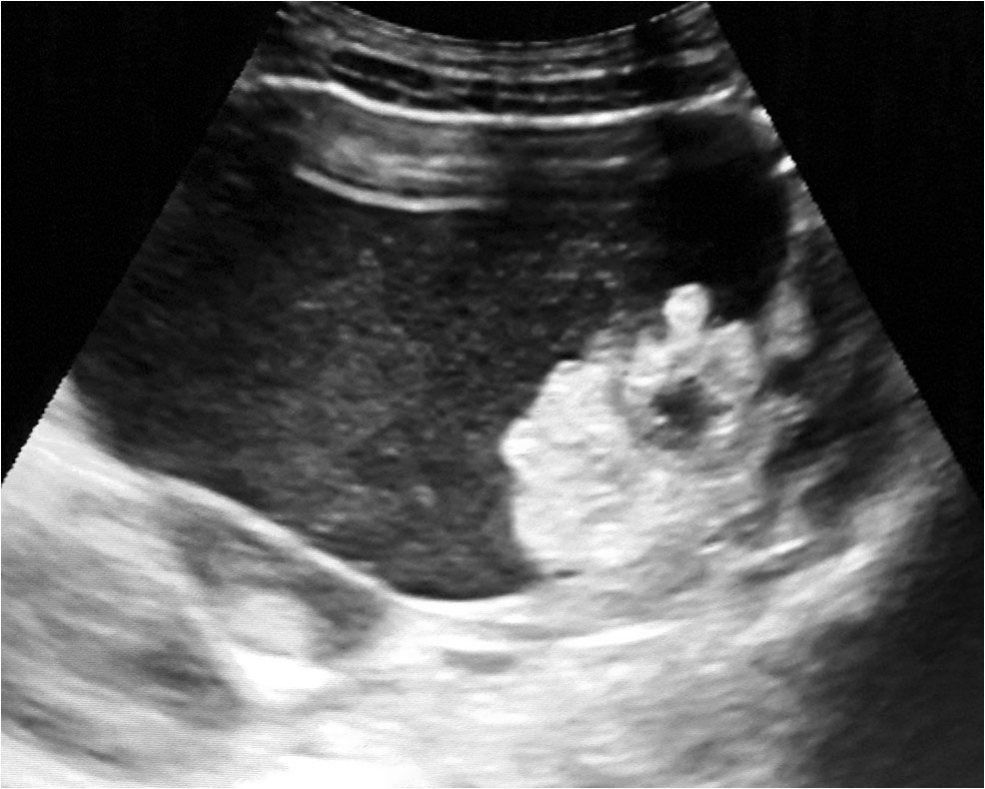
Transverse sonogram of a 20-year-old female with a mature cystic teratoma. A densely echogenic protuberance on the left side of the wall projecting into the cystic lumen is consistent with a Rokitansky nodule
